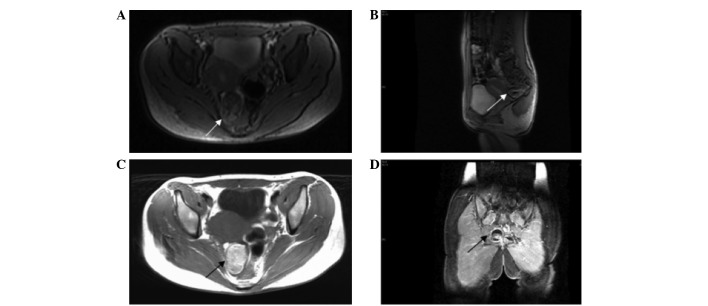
Enhanced magnetic resonance imaging (MRI) of the pelvis. (A) A transverse section of the mass (arrow) in a T1WI. (B) A sagital section of the mass (arrow) in a T1WI, posterior to the uterus and approaching the sacrum. (C) A transverse section of the mass (arrow) in aT2WI. (D) A coronal section of the mass (arrow) revealed that the mass was located in the right region of the pelvic cavity, at the right side of the rectum.
